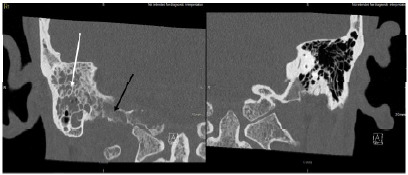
Coronal view computed tomography temporal/petrous bones re-demonstrating right mastoid air cell opacification (white arrow) along with aggressive osseous erosions of the occipital condyle and skull base (black arrow) with reference normal-appearing left-sided mastoid air cells, occipital condyle, and skull base.
